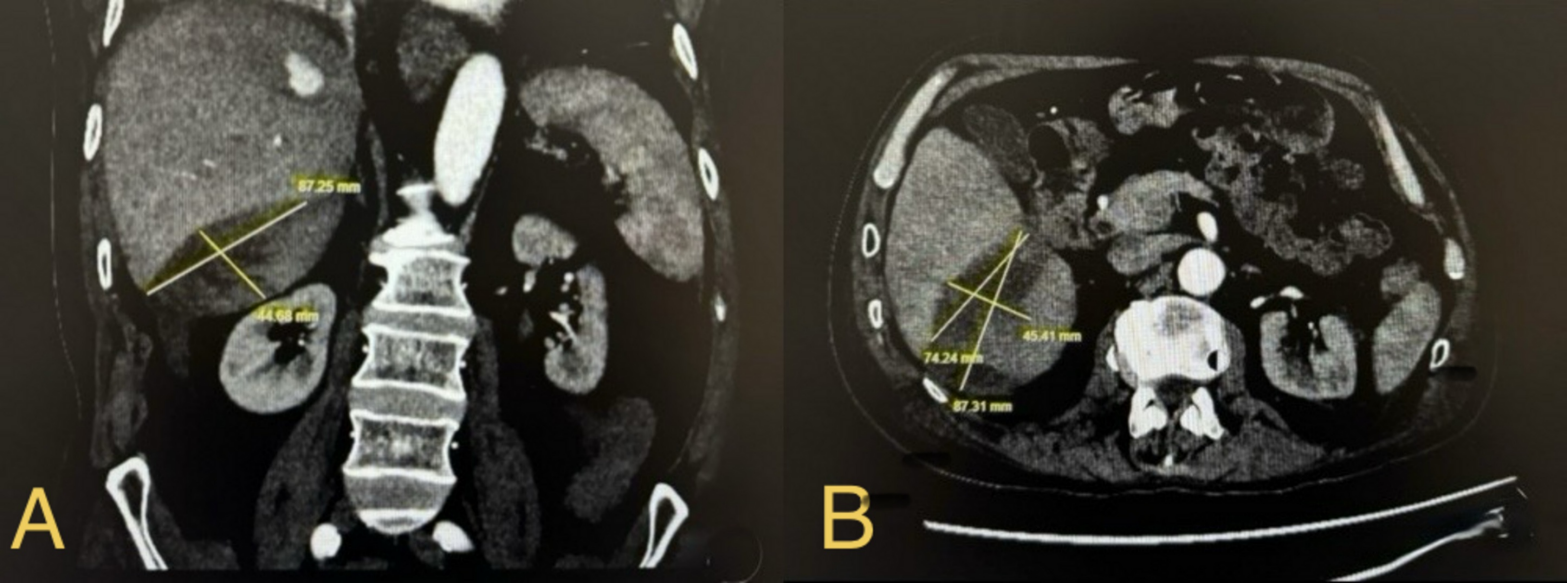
Contrast-enhanced CT scan of the abdomen showing a large right hepatic subcapsular haematoma: (A) coronal and (B) axial views
